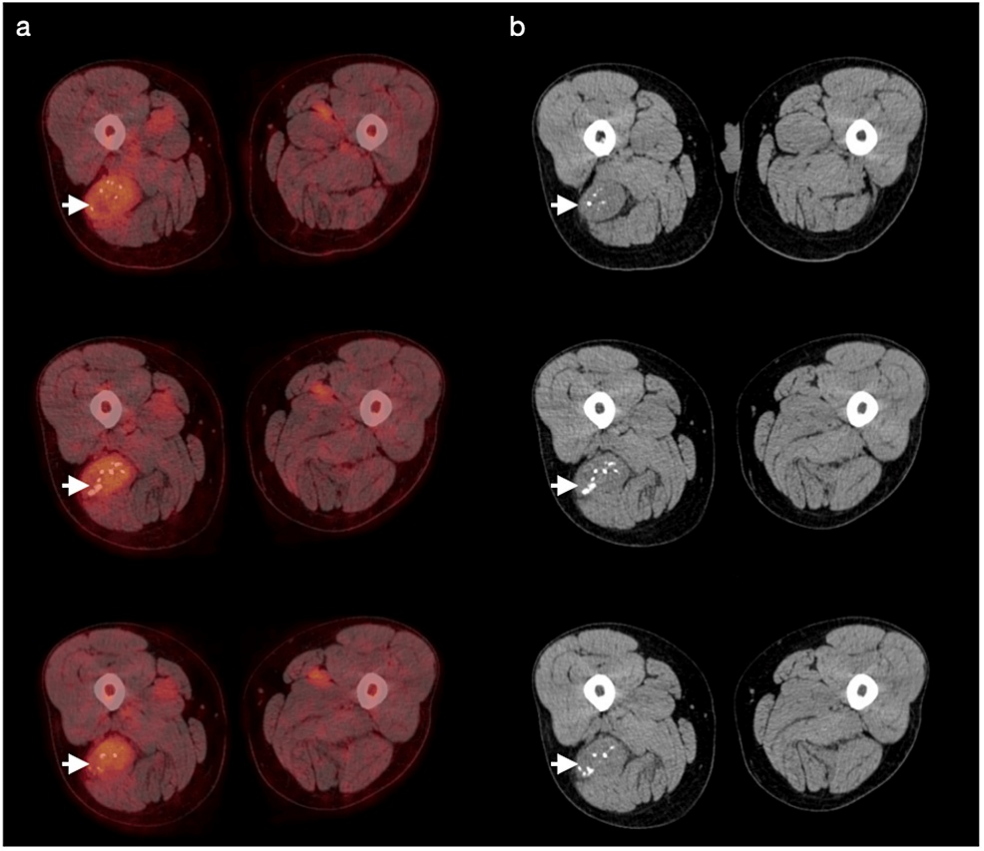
Axial images from proximal to distal (from top to bottom, respectively) showing a posterior, lateral, hypermetabolic thigh mass with extensive internal calcifications (a) Positron emission tomography. (b) Computed tomography
